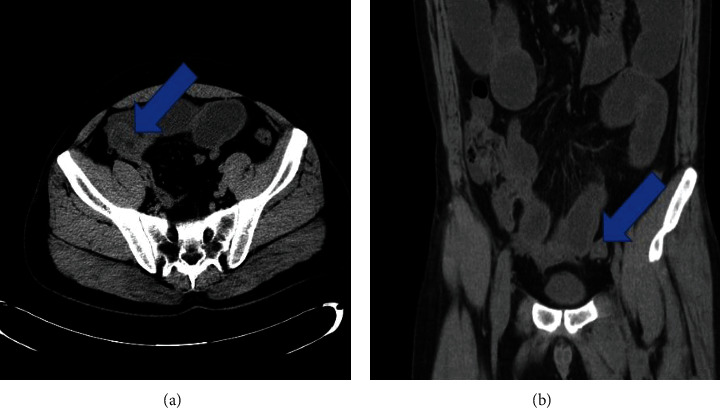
CT image of patient's intestinal obstruction: front view (a); side view (b). The images depict the location of the patient's intestinal obstruction.
